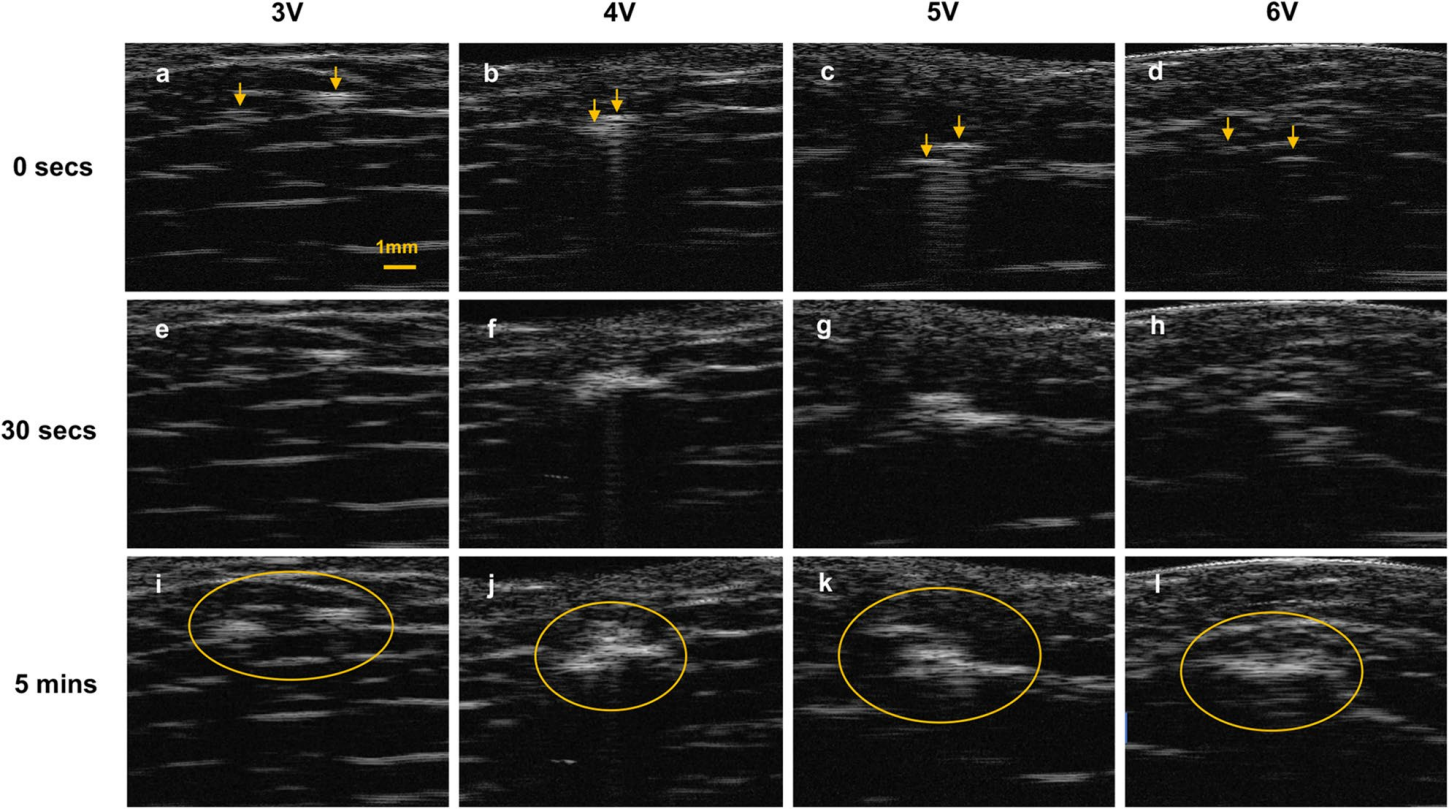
High frequency ultrasound images of adipose samples following ECLL. Adipose samples were imaged using high frequency ultrasound at (a–d) 0 s (secs) of ECLL, (e–h) 30 s of ECLL, and (i–l) 5 min of ECLL. Images of increasing voltage are displayed from left to right. Yellow arrows indicate electrodes. Yellow circles surround the hyperechoic region of gas formation.
